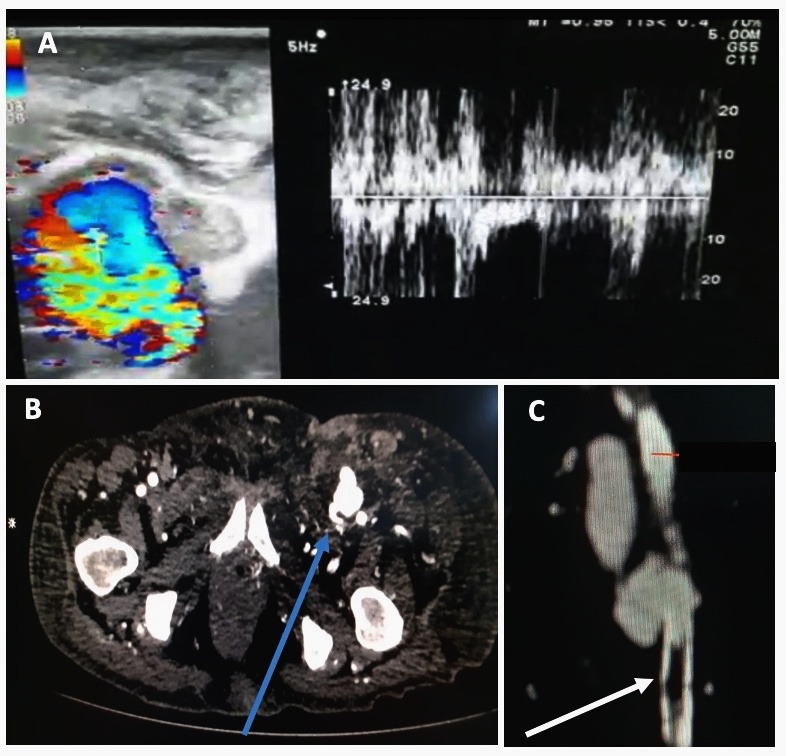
(A) Duplex ultrasonography demonstrating the characteristic turbulent spectral pattern of an arteriovenous fistula (AVF); (B) Computed tomography angiography (axial) demonstrating the presence of high-density contrast in femoral vein suggestive of an AVF (blue arrow); (C) Computed tomography reconstructed image of left superficial femoral artery (SFA) indicating the presence of thrombus in the SFA (white arrow).
